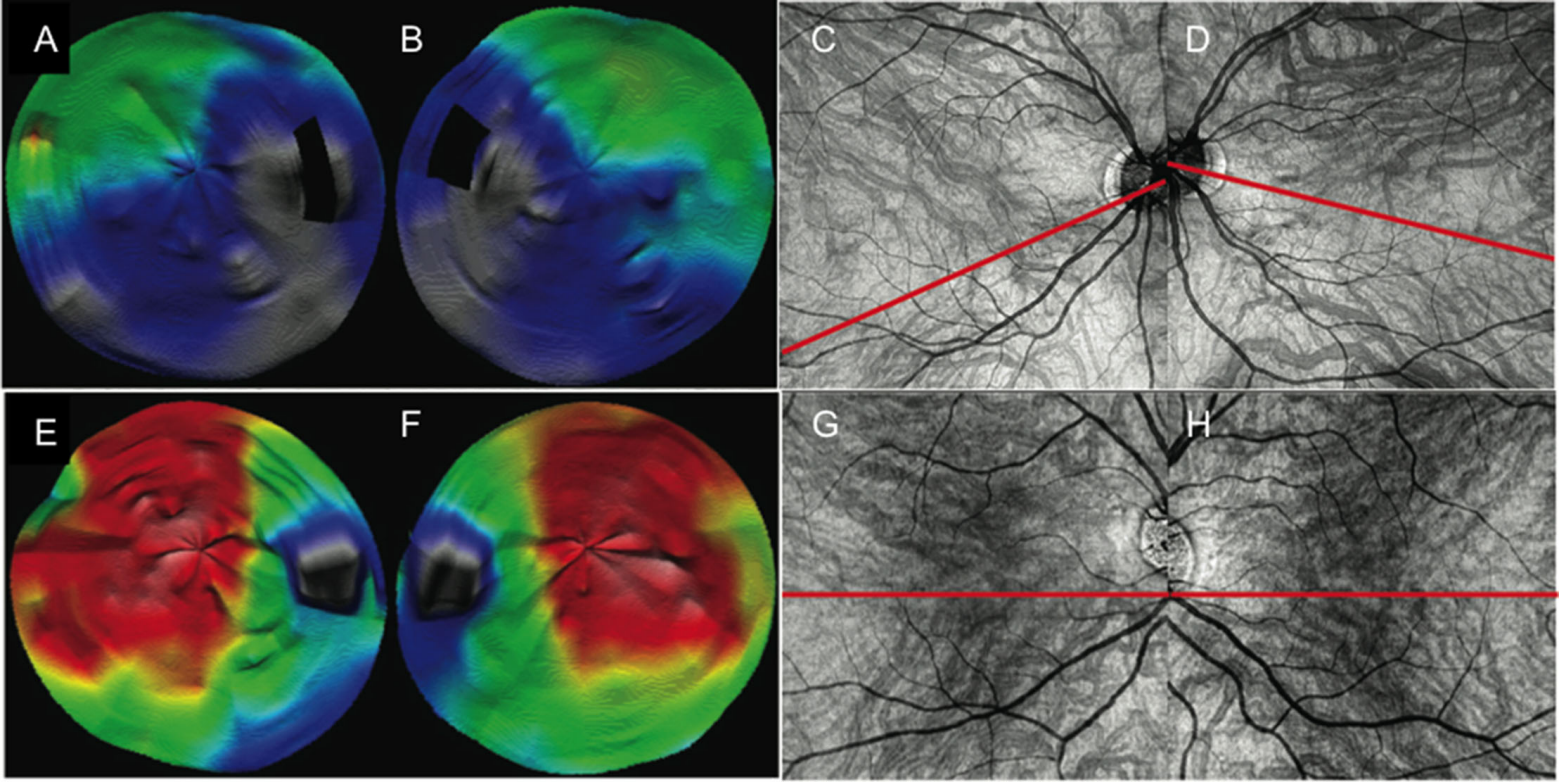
Correspondence between choroidal thickness maps and topography of choroidal veins on en face OCTA images. Choroidal thickness (CT) maps obtained from 12-mm radial scans: (A, B) medium-thin choroids and (E, F) thick choroids); corresponding en face OCT-A images of the large choroidal vessels: (C, D) medium-thin choroids and (G, H) thick choroids. Asymmetrical CT patterns (A, B) are characterized by an asymmetrical distribution of the choroidal veins, with the presence of oblique watershed zones with respect to the horizontal axis (C, D). Symmetrical CT patterns (E, F) correspond to a symmetrical distribution of these veins with respect to the horizontal axis (G, H). Color code: red, >400 µm; yellow, 300–400 µm; green, 200-300 µm; blue, <200 µm.
